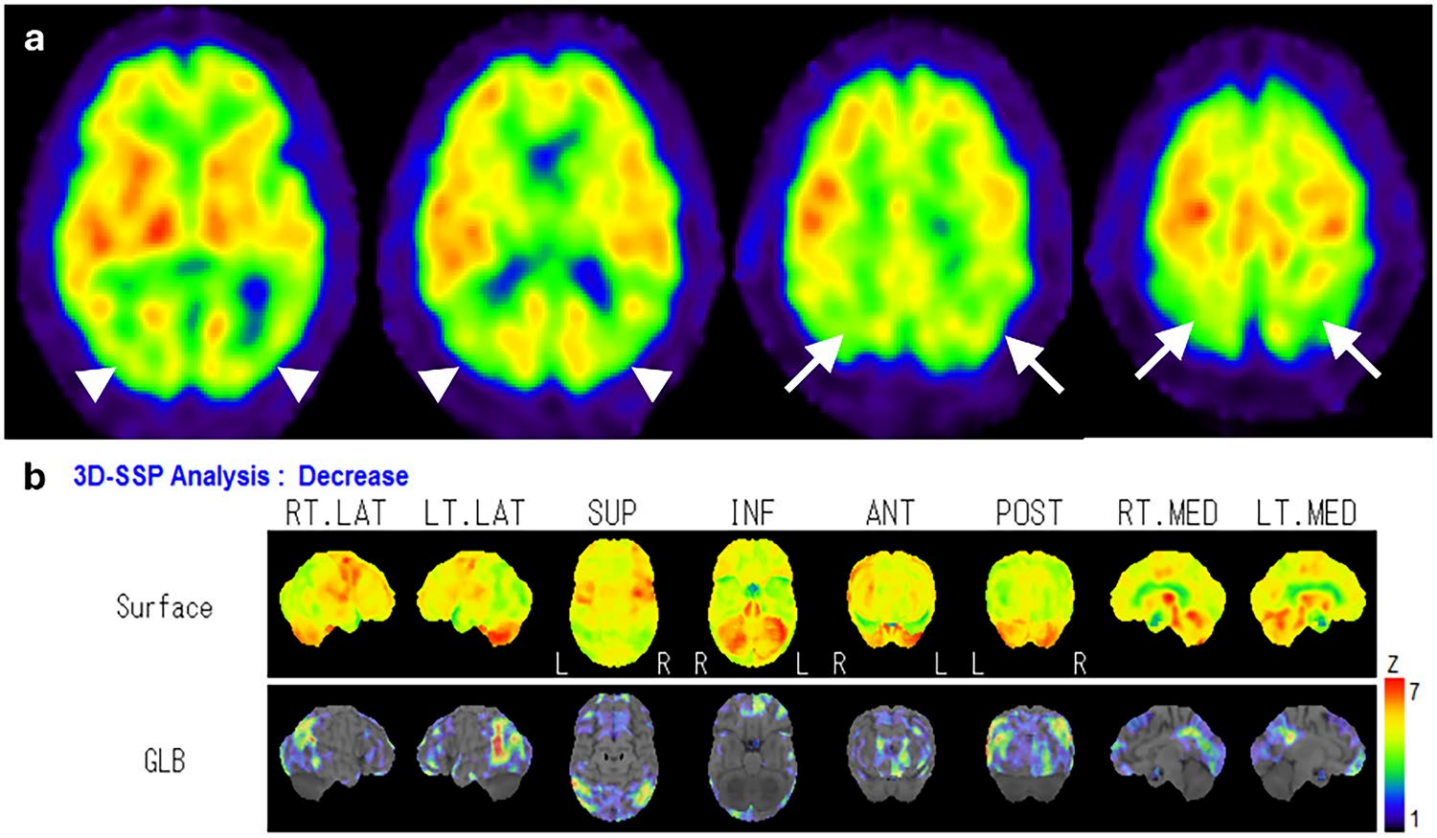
A representative case of DLB, a 69-year-old male presented with cognitive impairment, visual hallucinations, and parkinsonism. There was decreased striatal accumulation on ^123^I-iofulupane SPECT and decreased myocardial accumulation on ^123^I-MIBG scintigraphy (not shown). a IMP brain perfusion images and b 3D-SSP analysis revealed hypoperfusion in the occipital lobe (arrowheads) in addition to the posterior cingulate gyrus to precuneus and temporoparietal cortex (arrows), consistent with DLB. The decreased accumulation in the posterior cingulate gyrus was evident, suggesting the possibility of concomitant AD pathology
